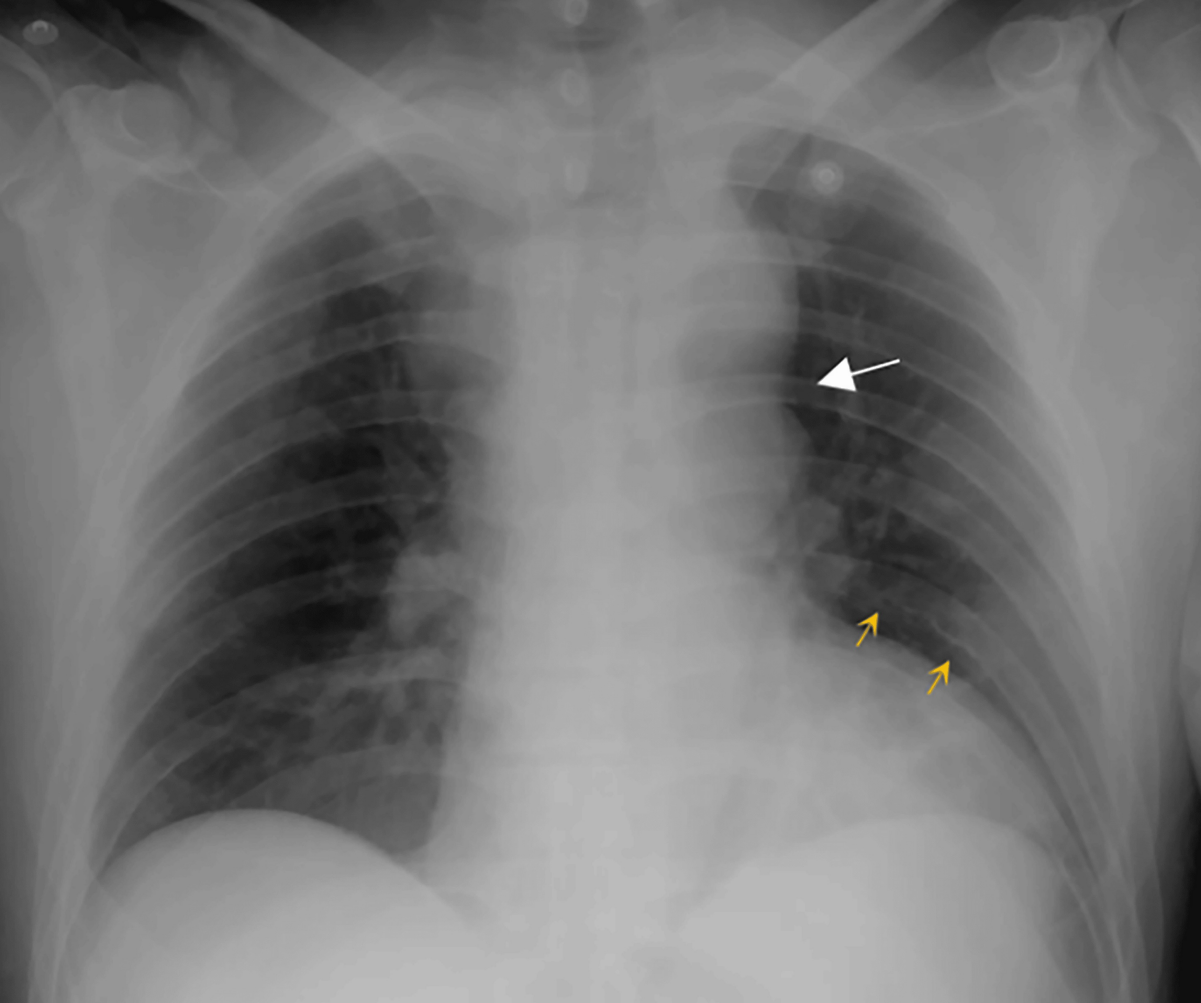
Posteroanterior chest radiograph showing the “figure 3 sign,” characterized by an indentation along the contour of the thoracic aorta due to a focal coarctation with pre and poststenotic dilatation (white arrow). Rib notching is also noted bilaterally (yellow arrows), representing collateral circulation through the intercostal arteries. This finding, known as “Roesler’s sign,” is caused by erosion of the inferior borders of the ribs secondary to increased flow through enlarged collateral vessels.
